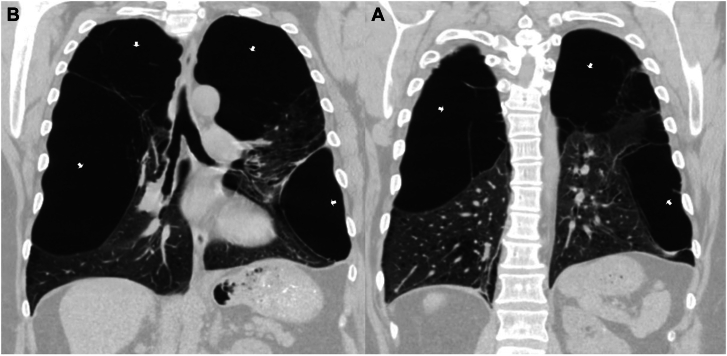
A, B, CT scan demonstrating giant bulla occupying greater than one-third of hemithorax. A, Axial slices from superior (left) to inferior (right) demonstrating bulla occupying lesions, highlighted by white arrows. B, Coronal slices from anterior (left) to posterior (right) demonstrating bulla occupying lesions, highlighted by white arrows.
